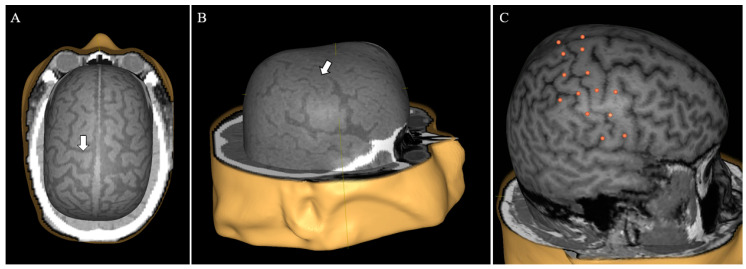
Head model based on computed tomography (CT). By using appropriate preprocessing, CT can serve as a viable basis for head model reconstruction in the nTMS system (A,B). Important anatomical structures such as the precentral gyrus are easy to identify (A,B, white arrows). For image testing, 12 targets were placed around the central sulcus on each hemisphere with two additional targets at the midline vertex (26 targets total per brain, C).
